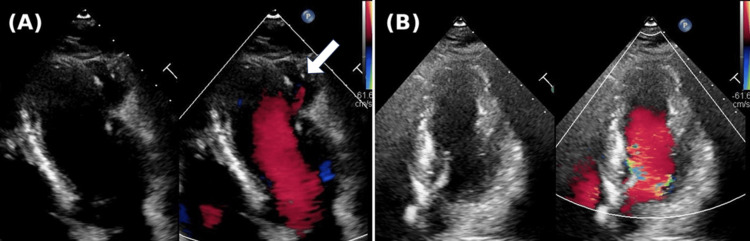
Echocardiographic images showing the left ventricle in diastole (A) Preoperative echocardiography. Blood flow entering the pseudoaneurysm is observed (white arrow). (B) Postoperative echocardiography. The pseudoaneurysm is completely sealed, and no leakage is detected.
